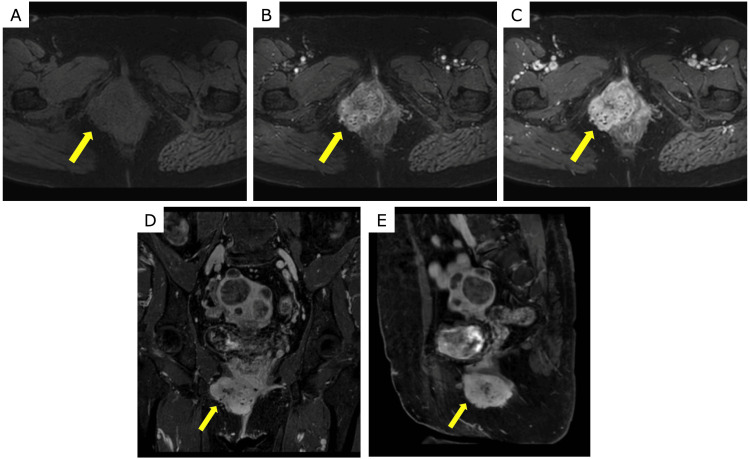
Dynamic contrast-enhanced and post-contrast images (A-C) Fat-suppressed dynamic contrast-enhanced T1-weighted images (A, pre-contrast; B, 30 s post-injection; C, 120 s) show a gradual enhancement pattern. (D, E) Fat-suppressed post-contrast T1-weighted images in the coronal (D) and sagittal (E) planes show that the mass has lobulated margins and abuts the right vaginal wall, without definite invasion of the bladder, rectum, or pelvic floor musculature
